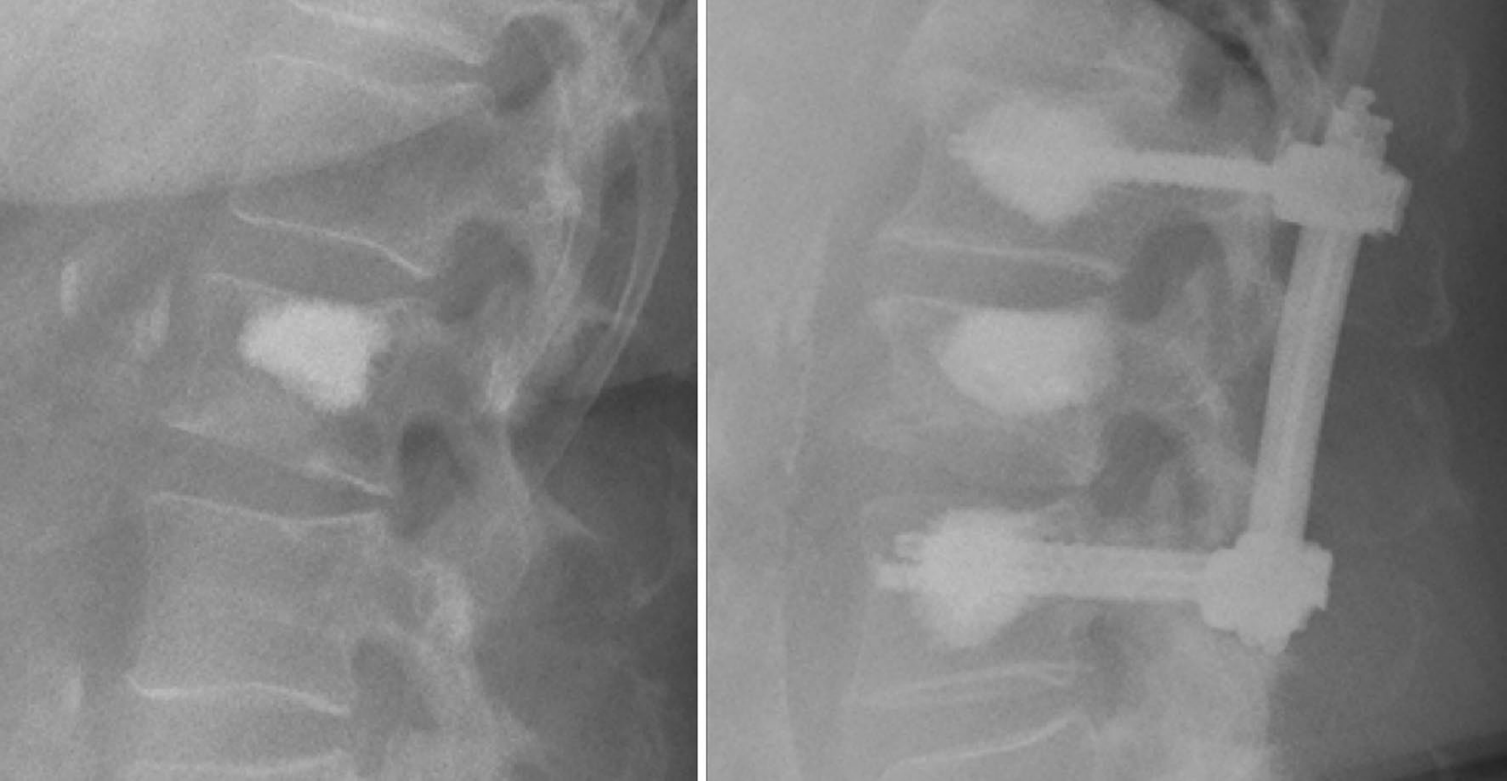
Sagittal X-ray of the lumbar spine. Left: vertebral cement augmentation (VCA); right: transcutaneous cement-augmented screw fixation with vertebral cement augmentation (HTSF)
